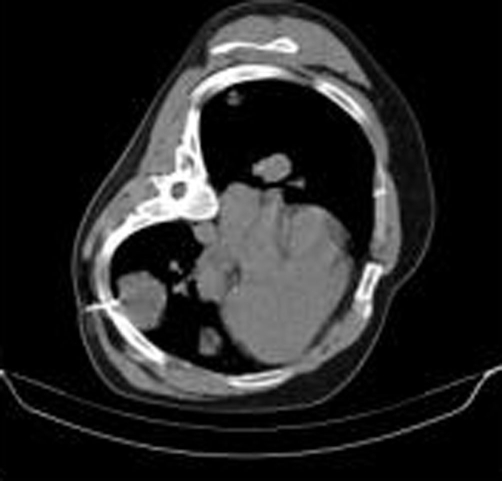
Chest non-enhancing CT scan, mediastinal window: the cutting needle was inserted in the chosen point. Patient was placed in left decubitus position in order to achieve the right pathway to the chosen lesion to be biopsied. The tip of the needle is seen inside the lesion.
